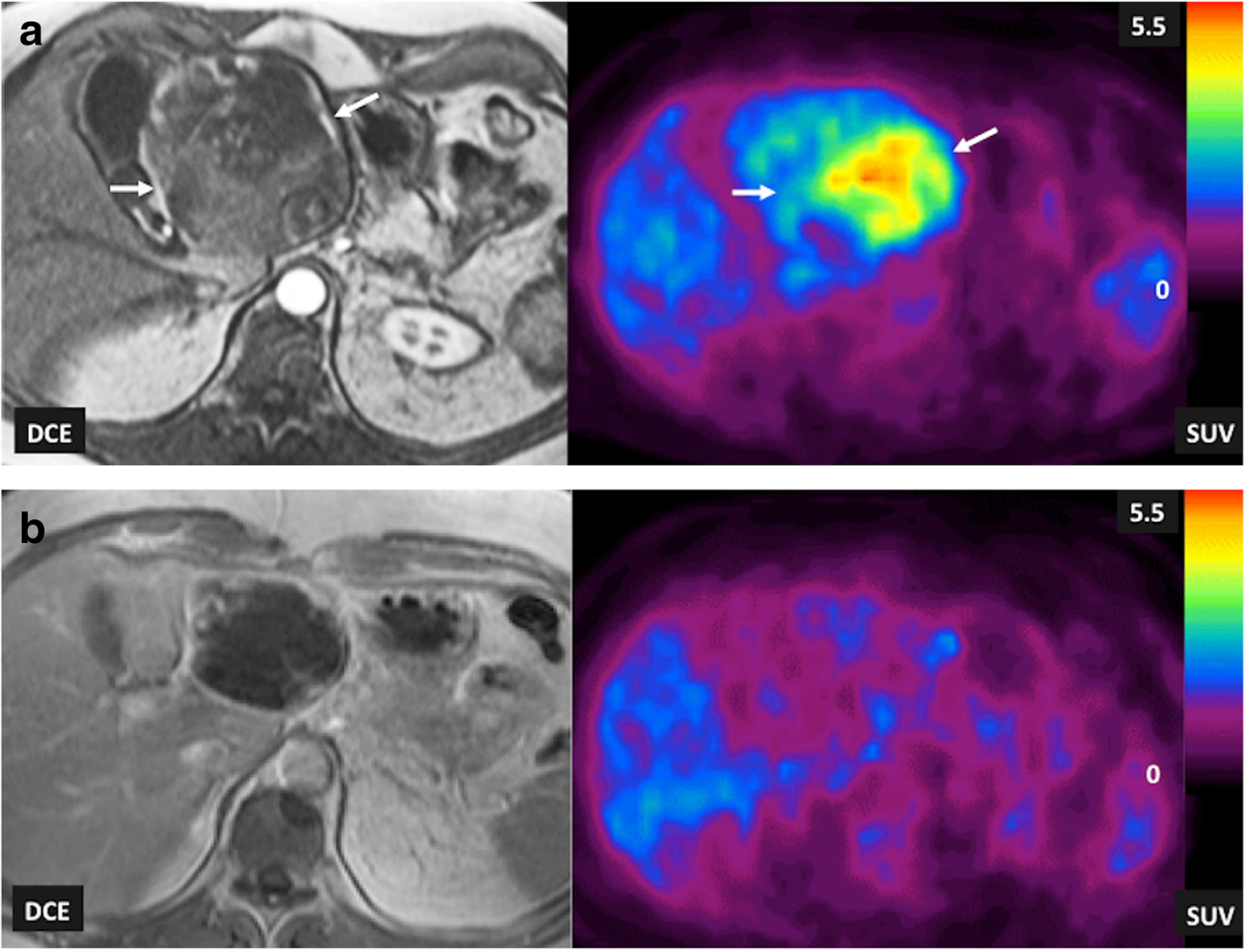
Liver metastasis of a gastrointestinal stromal tumor (GIST) in a 68-year-old man. T1-weighted contrast-enhanced MRI (DCE) images and FDG-PET SUV parametric maps (SUV) acquired pretherapy (a) and following the administration of imatinib (b) and pretherapy (a), imaging findings evidenced a metabolic active tumor with areas of heterogenic enhancement (white arrows). Post-therapy (b), the tumor presented almost a total absence of enhancement and a complete metabolic response on PET imaging
